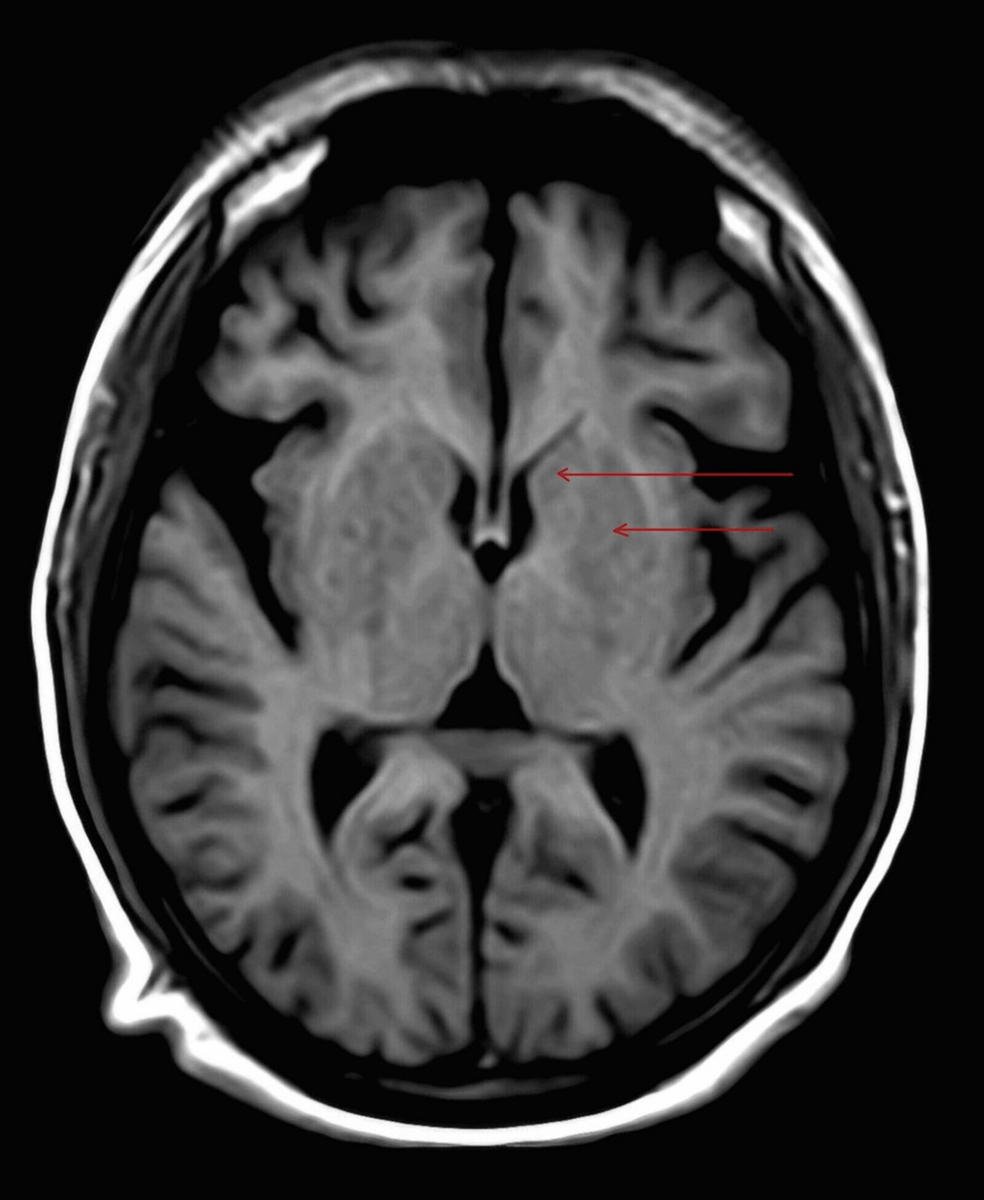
This shows an MRI T1-weighted image with no obvious abnormality in the basal ganglia (red arrows showing basal ganglia)
